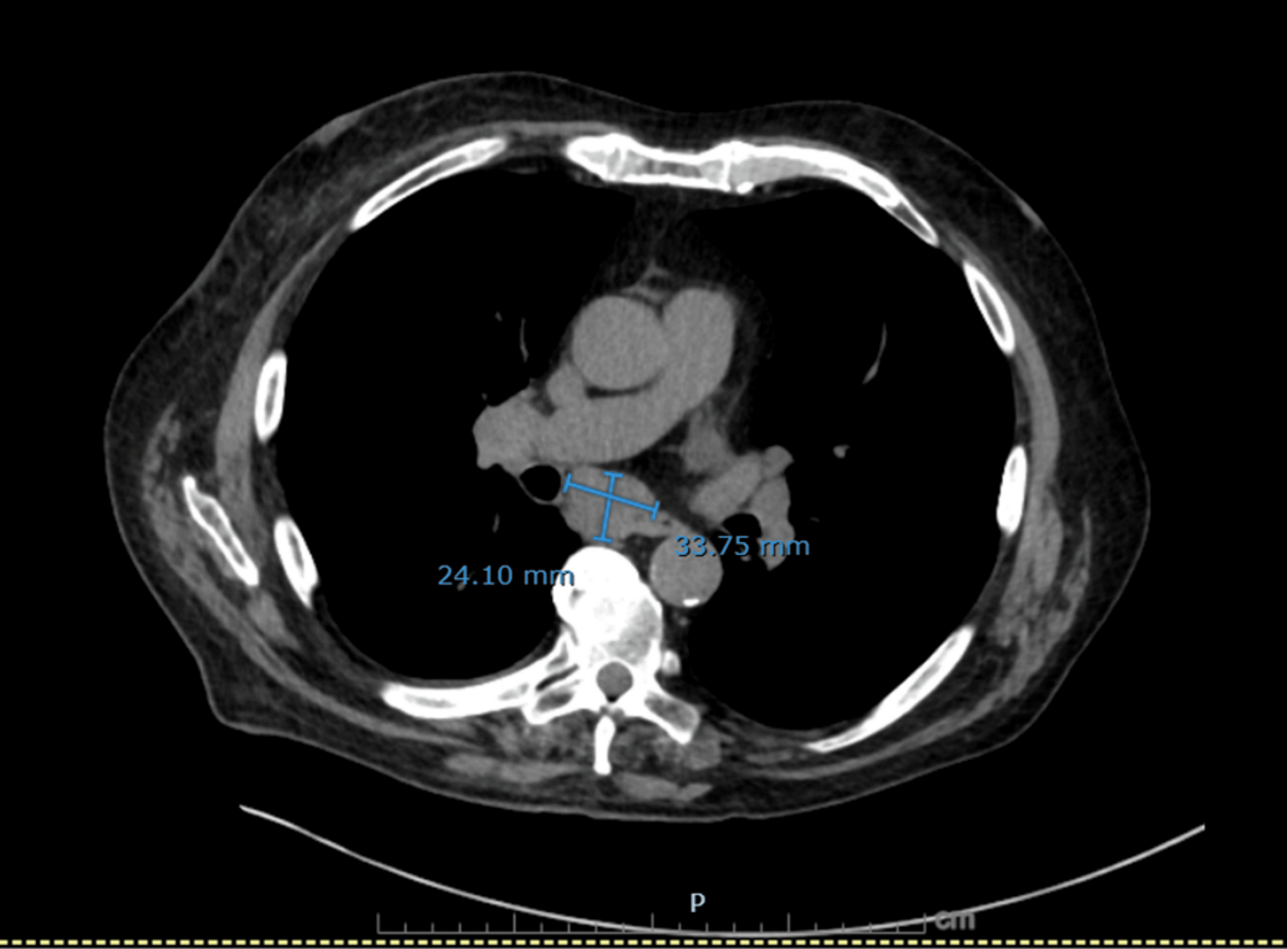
Axial non-contrast CT chest showing markedly enlarged subcarinal lymph node A dominant subcarinal lymph node measuring 24 × 34 mm, highly suspicious for malignant mediastinal involvement.
